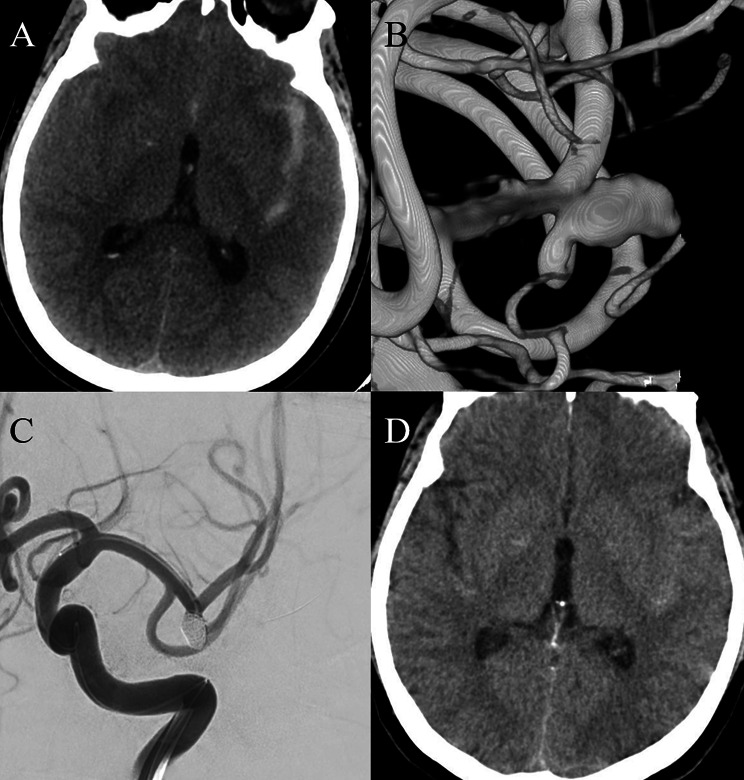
Computed tomography (A) and digital subtraction angiogram (B) revealed subarachnoid hemorrhage (SAH) secondary to a ruptured middle cerebral artery aneurysm. Patent was managed with successful endovascular coil embolization of the ruptured aneurysm without stent assistance (C). Computed tomography scan 7 days after onset of symptoms showed near-complete resolution of the SAH (D)
